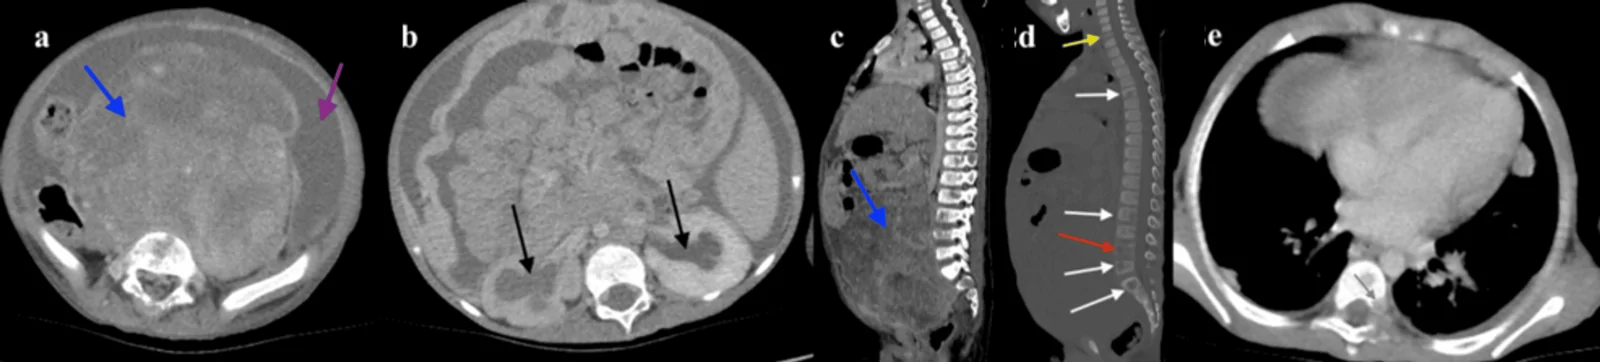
Initial contrast-enhanced abdominopelvic CT. (a) Axial image at the portal venous phase showing the large heterogeneous retroperitoneal mass (blue arrow) with a peritoneal effusion (purple arrow). (b) Axial image showing mass effect on adjacent structures with bilateral hydronephrosis (black arrows). (c) Sagittal reformation showing the cranio-caudal extent of the mass (blue arrow). (d) Sagittal bone-window CT image demonstrating multilevel lytic metastatic involvement of the lumbosacral spine (white arrows), with a pathologic compression fracture of L4 (red arrow). A C6 compression deformity is also present (yellow arrow), without a definite associated focal osseous lesion to establish its metastatic nature. (e) Axial bone-window CT image at the T5 level showing a lytic soft-tissue metastasis centered on the left pedicle, extending to the ipsilateral transverse process and vertebral lamina and into the spinal canal (brown arrow).
